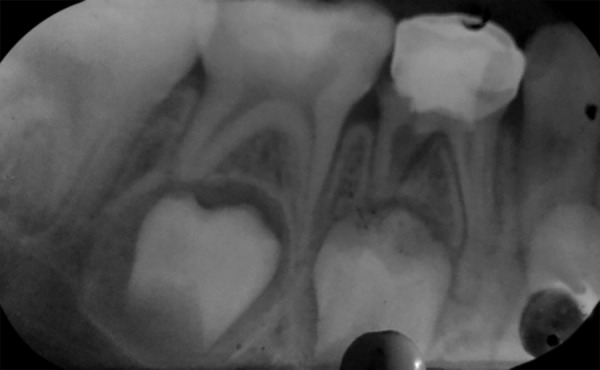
Radiographic failure in the form of internal resorption seen with calcium hydroxide pulpotomy
